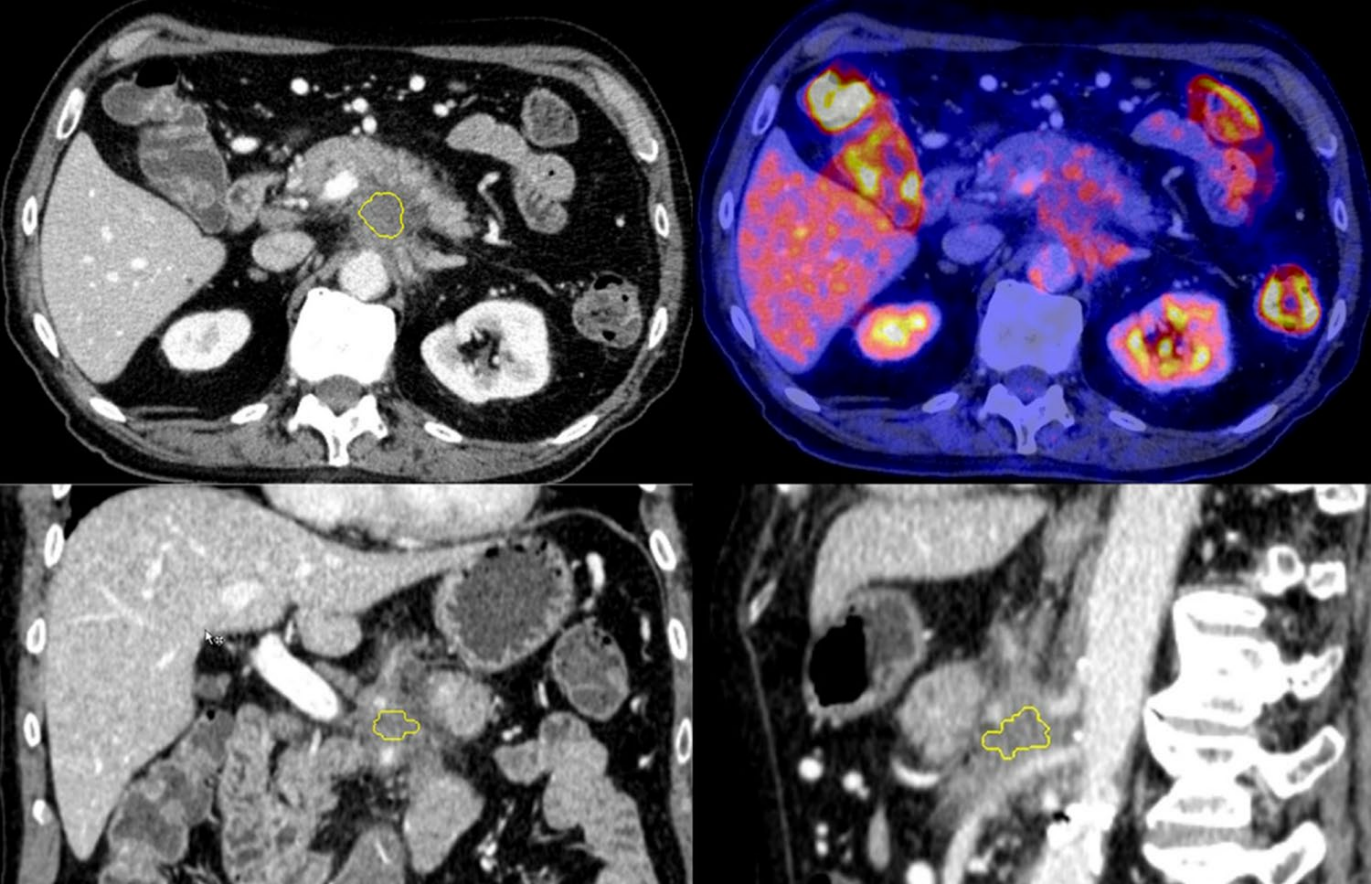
Tumor segmentation. A 80-year-old male patient with PDAC infiltrating the celiac trunk and showing only moderate tracer avidity in ^18^F-fluorodeoxyglucose (FDG) positron emission tomography/computed tomography (PET/CT) (right upper row). Three-dimensional tumor segmentation under exclusion of adjacent structures
